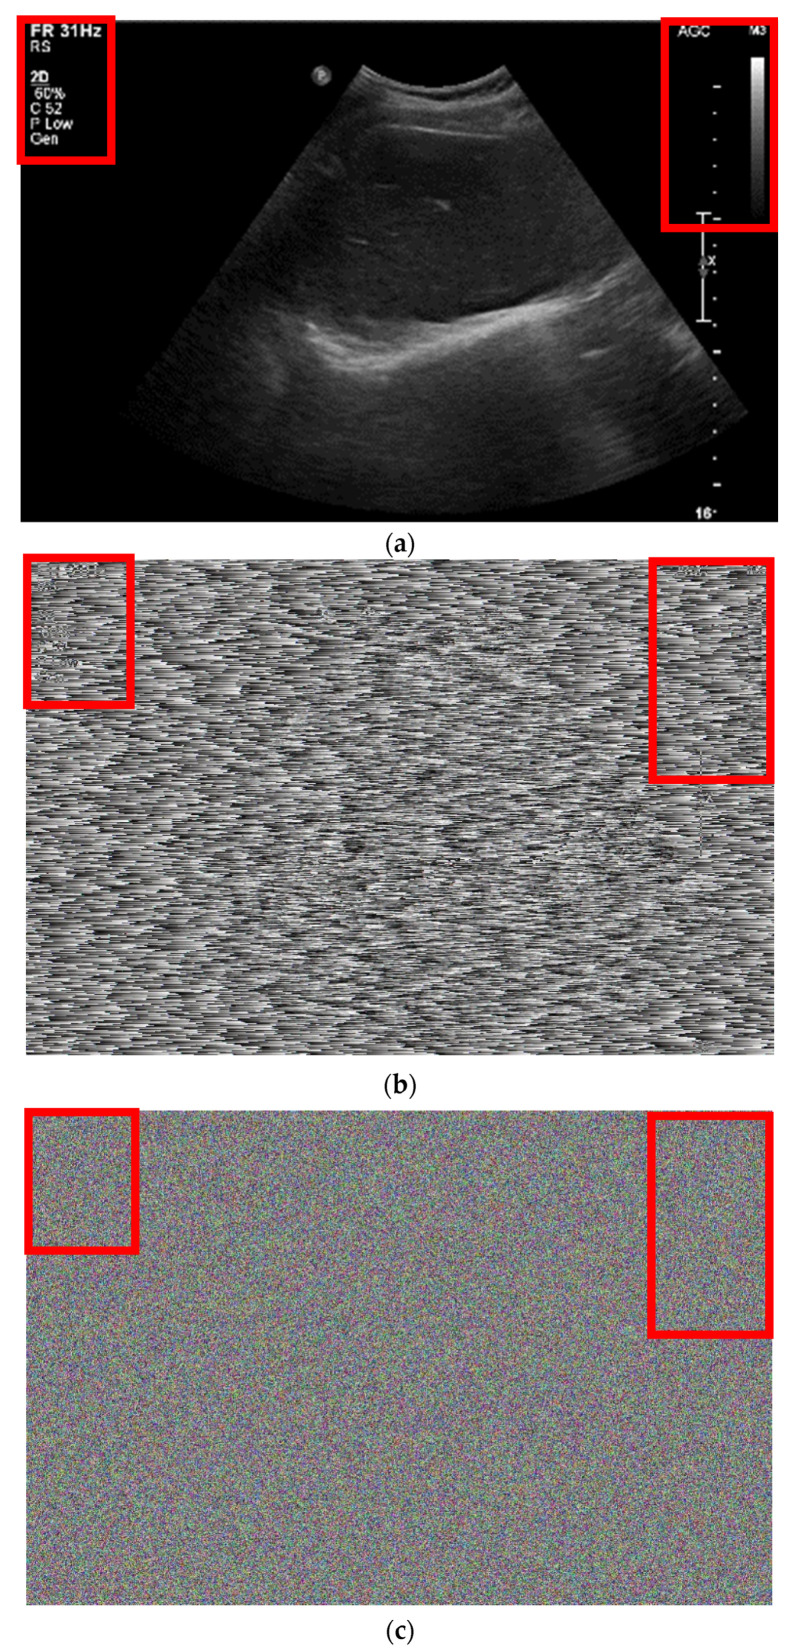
(a) Obtained original ultrasound image and enlarged images of (b) Figure 4i and (c) Figure 10i.
